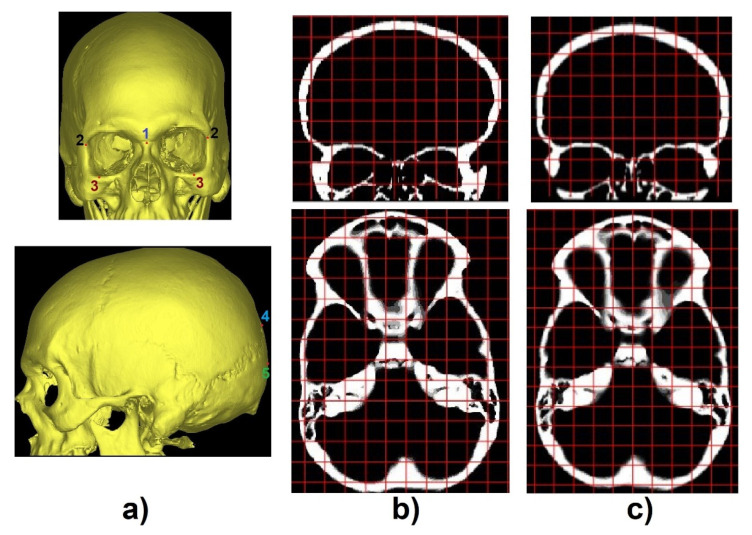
(a) Identification of cephalometric landmarks in coronal (top) and sagittal plane (bottom), (b) CT slices misaligned, and (c) CT slices after the alignment procedure (coronal plane-top and transverse plane-bottom).
